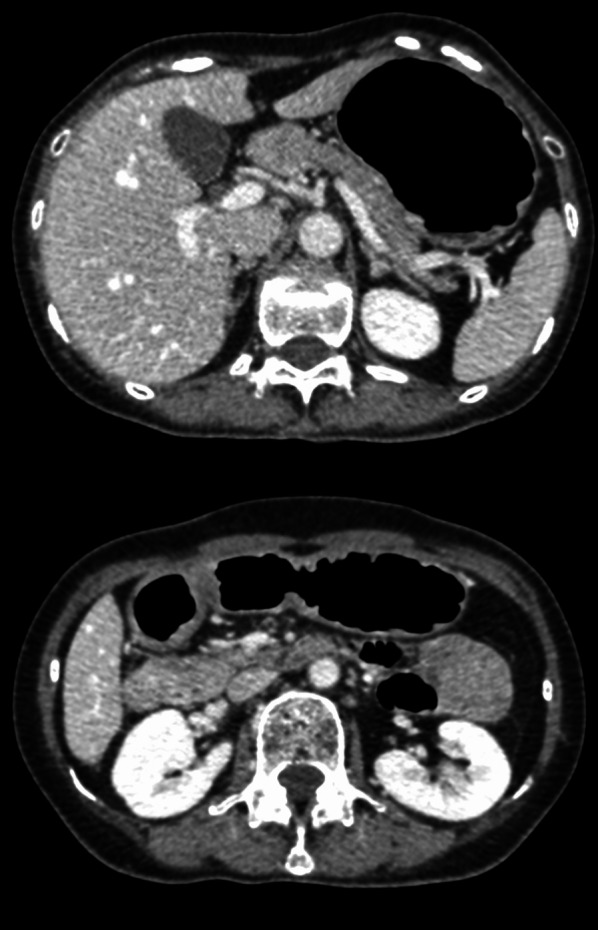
Contrast computed tomography image showed no swelling lymph nodes or distantmetastasis
